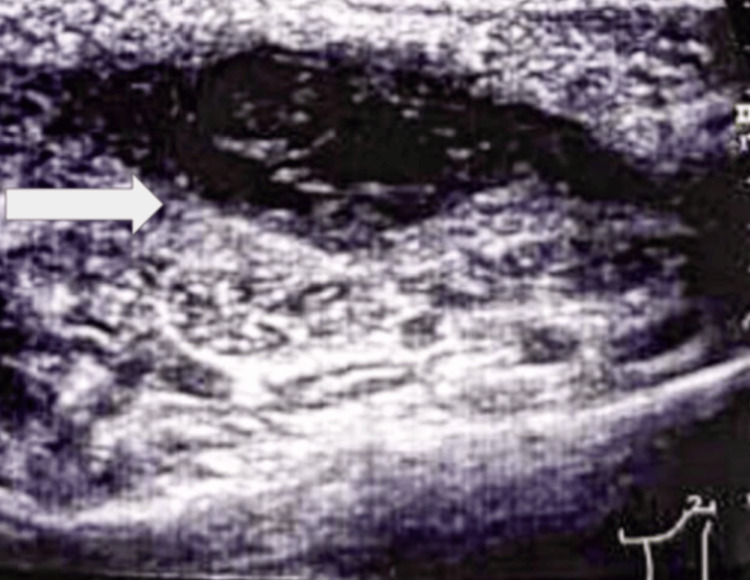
An ultrasound image of the neck (arrow) shows a space-occupying lesion of oval shape, parallel to the skin, with partially circumscribed margins, with heterogeneous echogenicity, measuring 26 x 22 x 11 mm, with a volume of 33 mm.
